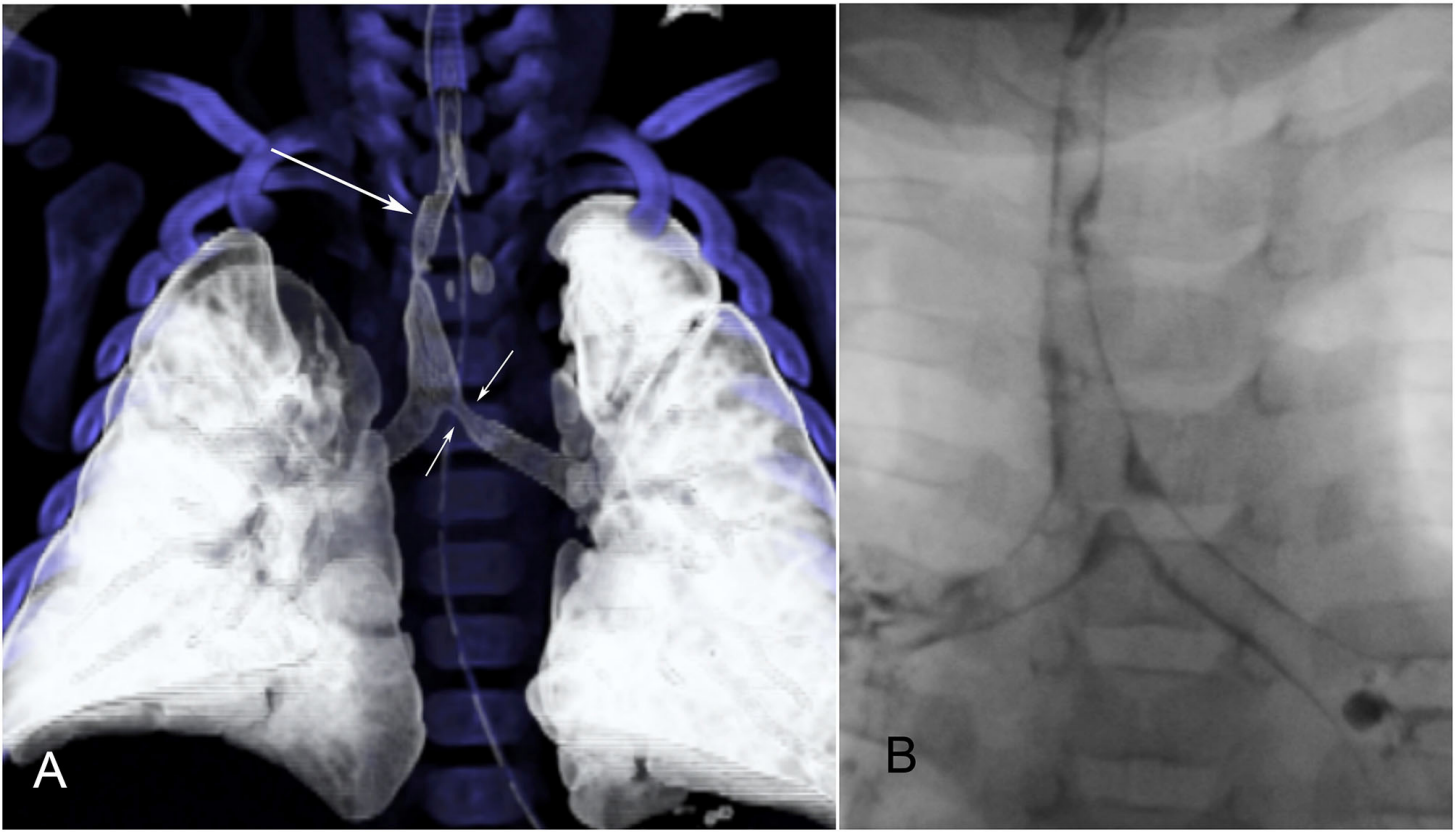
Congenital tracheal stenosis with bronchomalacia. (A) Reformatted CT image shows the congenital stenosis (long arrow) quite well. A second area of narrowing is present at the proximal end of the left bronchus (short arrows) on this single-phase study. (B) Dynamic bronchography shows that this is due to bronchomalacia and not a second stenosis.
